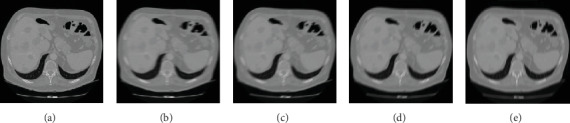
The results of different blurred angle of the liver tumour patient. (a) Original CT image. (b) Blurred angle of 0°. (c) Blurred angle of 30°. (d) Blurred angle of 60°. (e) Blurred angle of 90°.
